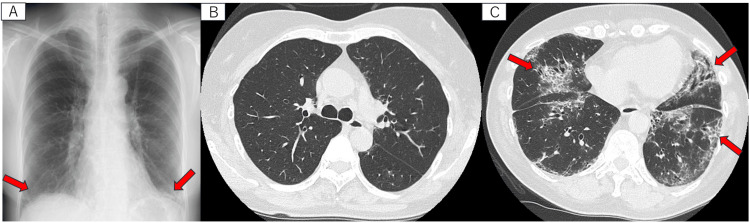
Chest X-ray and computed tomography before the bronchoscopy. There is ground-glass opacity in the bilateral lower lung fields (A). There are few lesions on the upper lobe (B). Both sides' middle and lower parts show ground-glass opacity, some with traction bronchiectasis. (C). Each red arrow indicates lesions associated with interstitial pneumonia.
